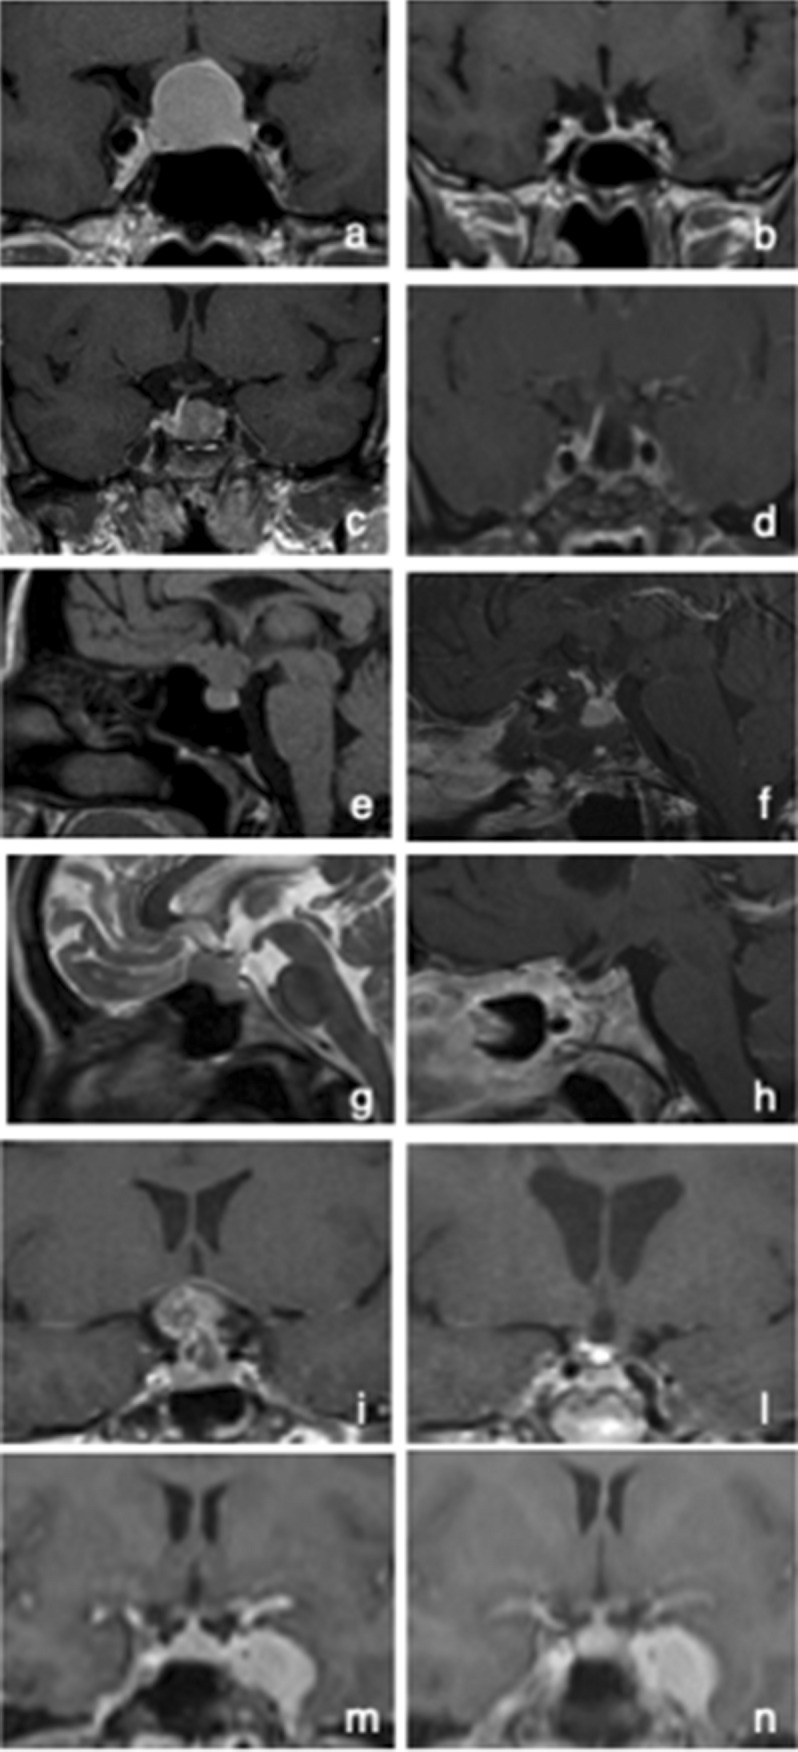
Coronal views of the gadolinium-enhanced T1-weighted pre- and post-operative MRI showing the present of an intrasellar pituicytoma (case #1) and ACTH-secreting macroadenoma (case #2) (a, c), and their complete exeresis (b, d). Sagittal view of the gadolinium-enhanced T1-weighted MRI showing a meningioma arising from the tuberculum sellae (e), removed through endoscopic endonasal approach, with the preservation of the pituitary gland and stalk (f) (case #3). Sagittal views of the pre-operative T2-weighted (g), and post-operative T1-weighted gadolinium-enhanced MRI (h), showing the complete exeresis of a tuberculum sellae meningioma (case #4). Sagittal views of the gadolinium-enhanced T1-weighted pre- (i) and post-operative MRI (l), showing a suprasellar craniopharyngioma and its total exeresis after the 2nd endoscopic endonasal surgery, respectively (case #5). Coronal view of the gadolinium-enhanced T1-weighted MRI showing a meningioma occupying the left cavernous sinus (m), and the results of hadronic radiation treatment (n)
